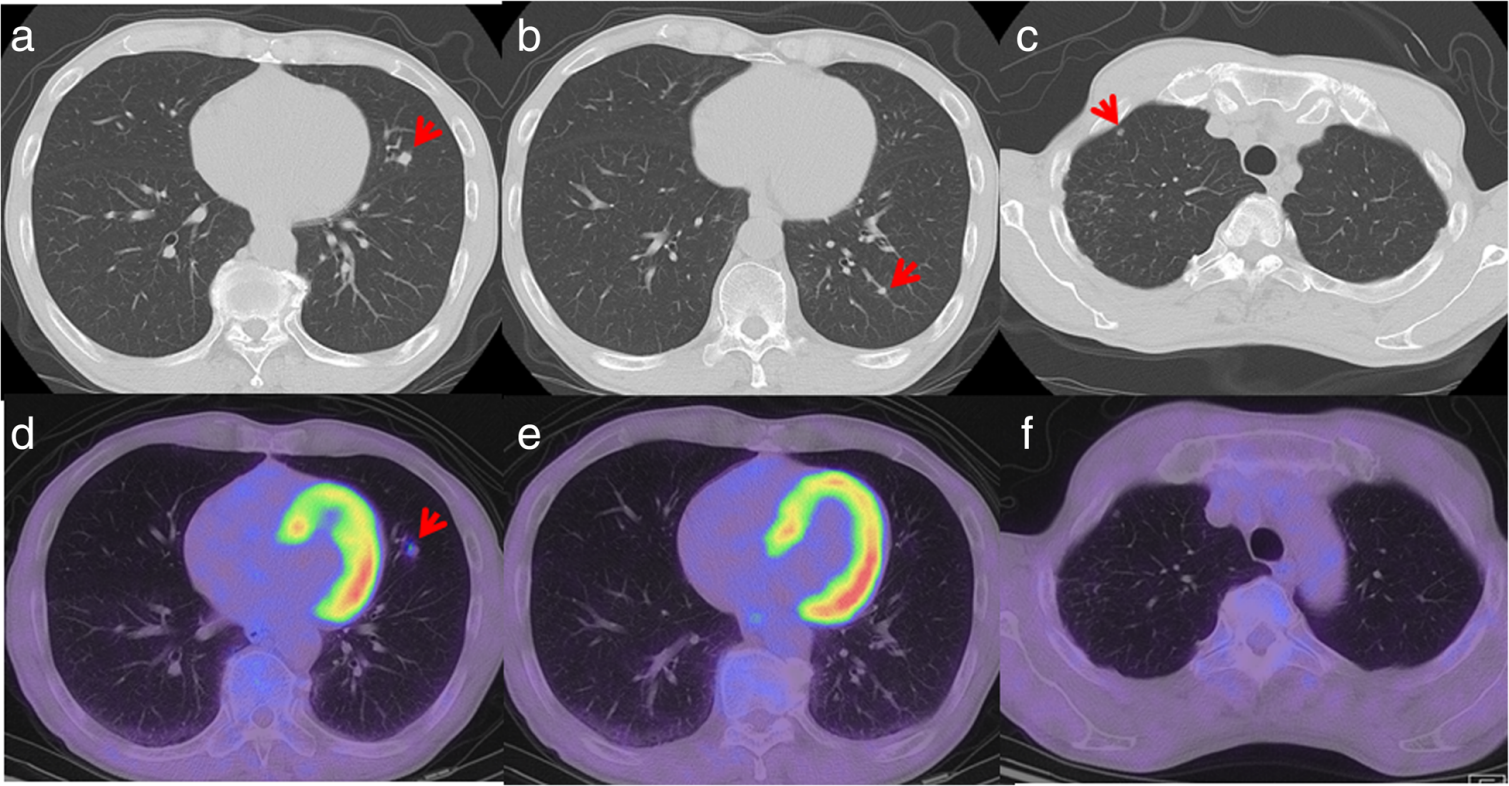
CT scan shows multiple nodes in S4 (a) and S10 (b) of the left lung and S1 (c) of the right lung. On PET-CT, abnormal FDG uptake is observed in S4 (d) of the left lung, but not in S10 (e) of the left lung or S1 (f) of the right lung (arrows)
